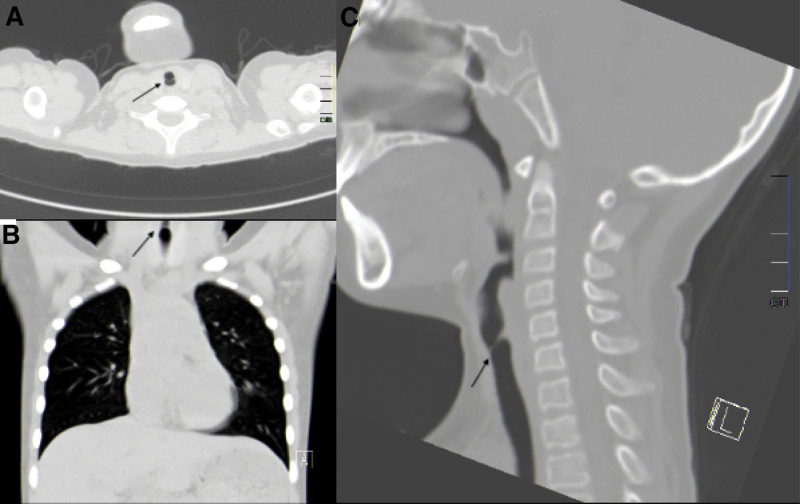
Axial (A), coronal (B), and sagittal (C) CT scan of the trachea depiction subglottic stenosis.
